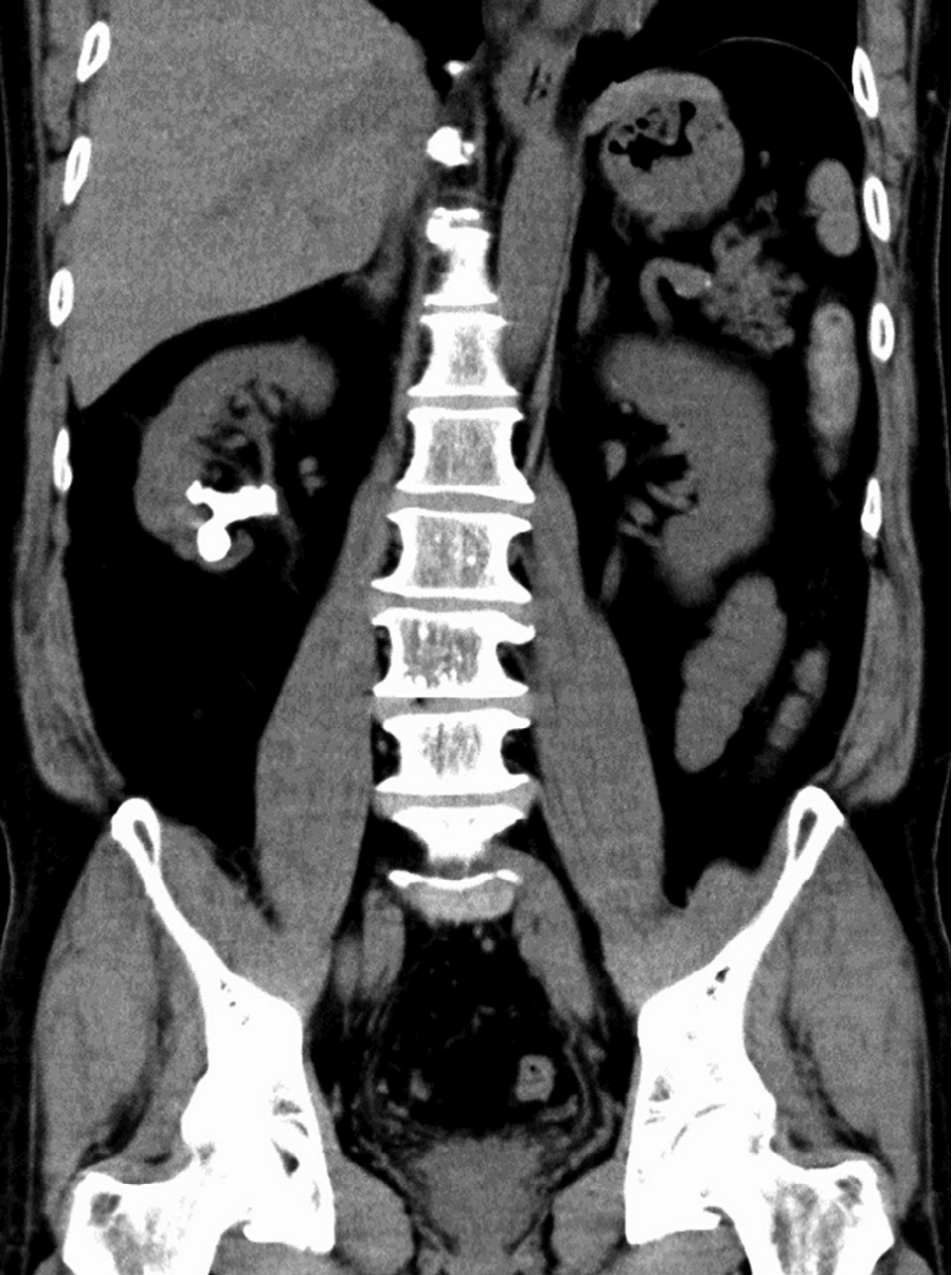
CT showing right middle and lower calyx stones (the longest diameter: 36 mm, the average CT value: 798 Hounsfield unit).
